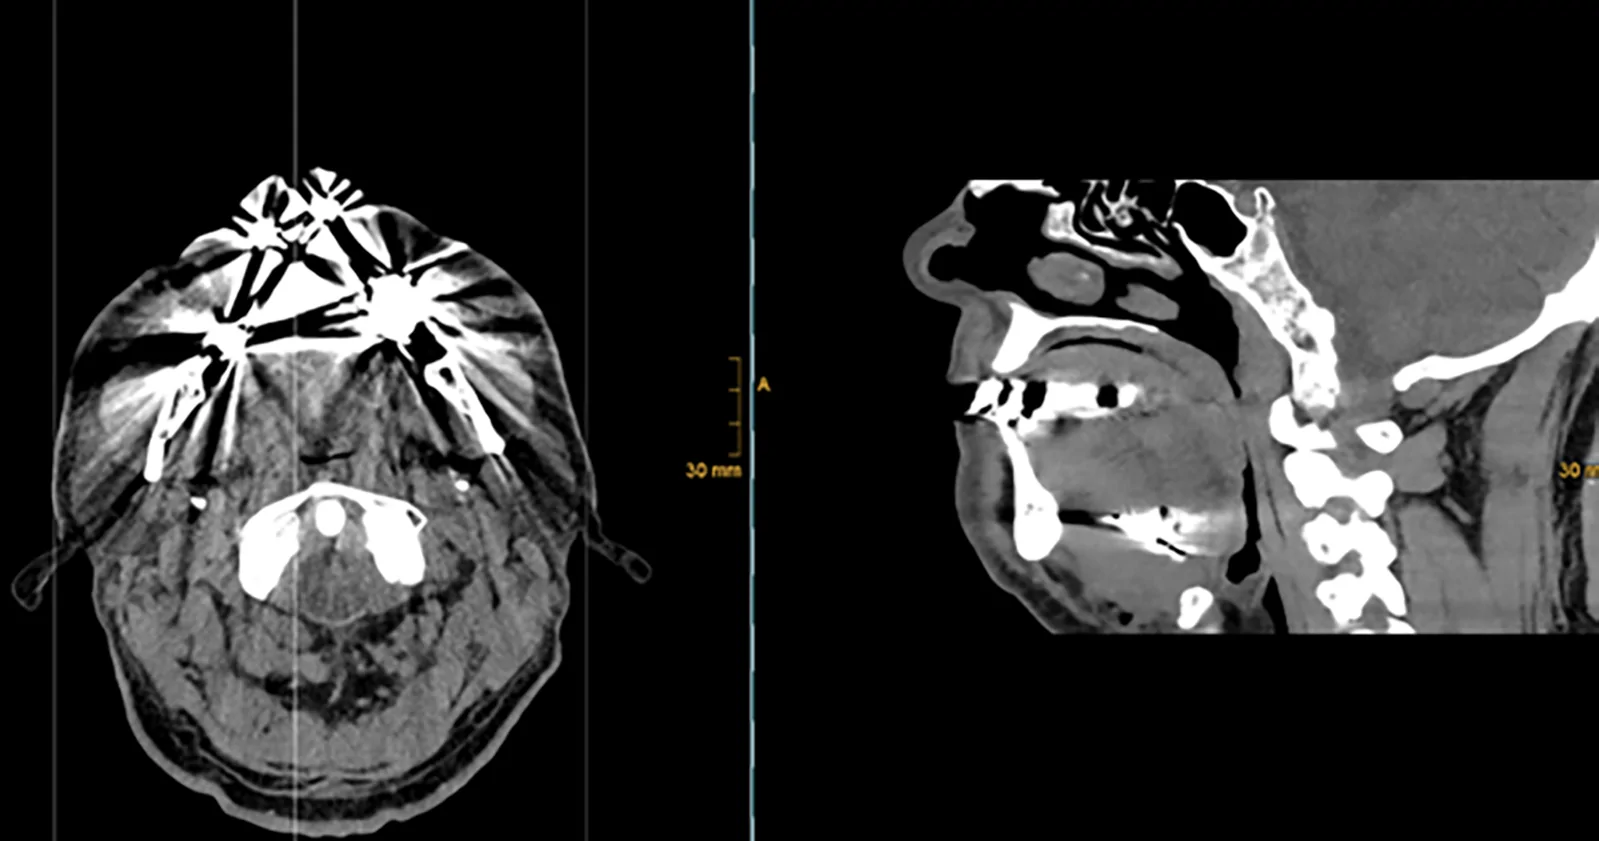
CT (axial and sagittal planes). biHGNS implant position and strong artifacts.
